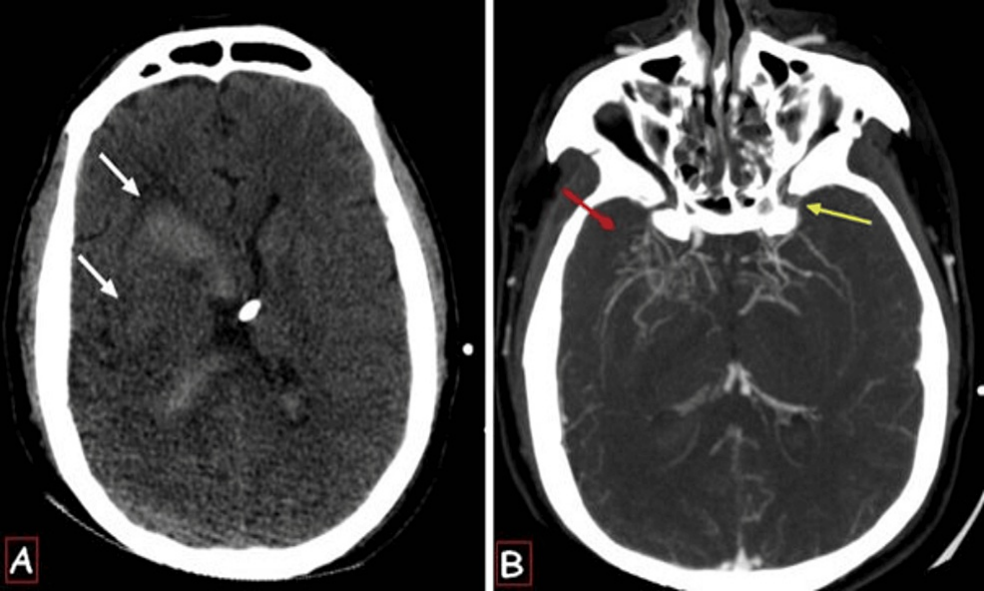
Axial brain non-contrast CT scan (A) showing a right capsulo-lenticular hematoma (white arrows) with ventricular flooding. CT angiography (B) showing the bilateral stenosis of the terminal portion of the internal carotid artery, middle and anterior cerebral arteries with a more pronounced lenticulostriate collateral network on the right side (red arrow), and abnormal collateral vessels from the branches of the external carotid artery on the left side (yellow arrow).
